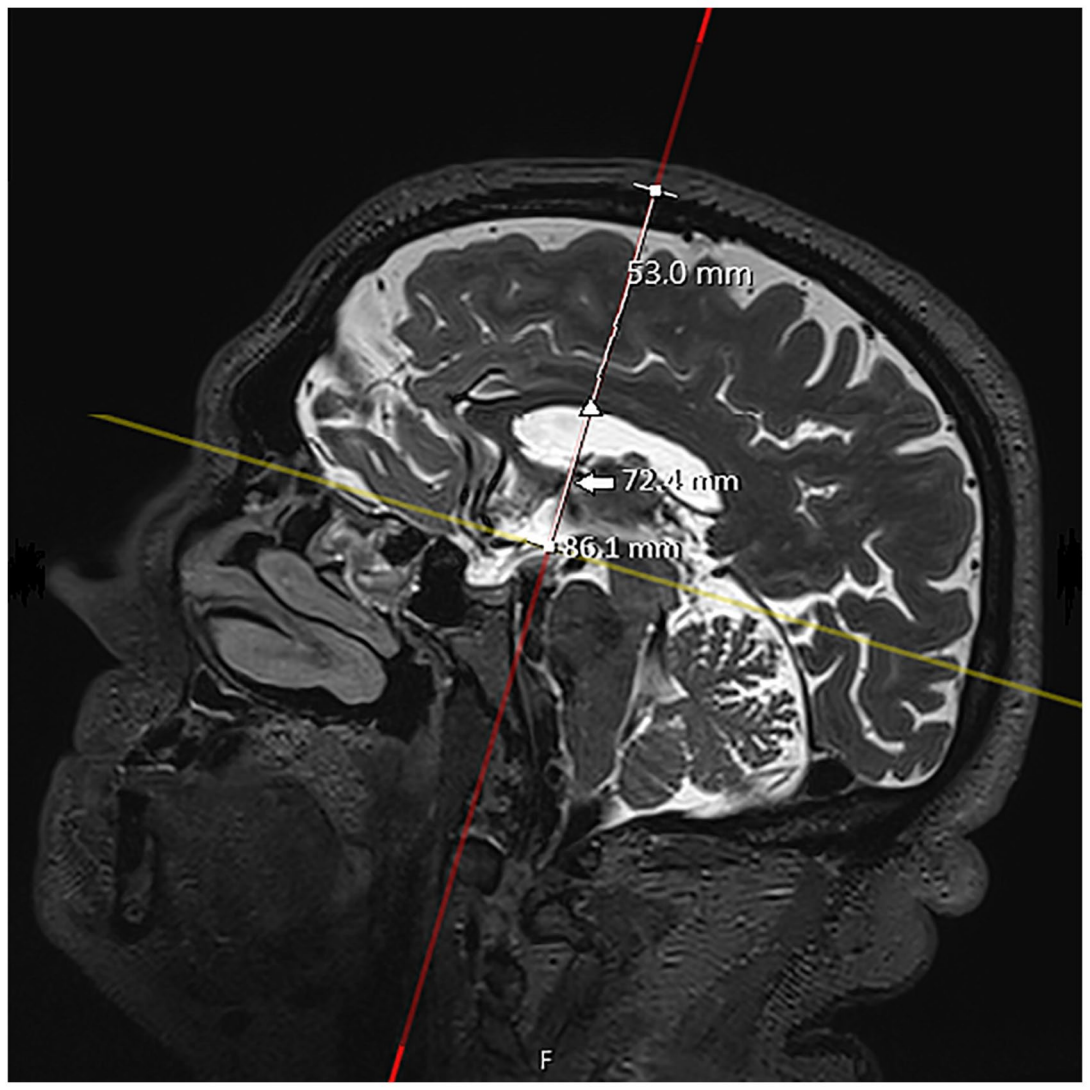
T2 SPACE MRI imaging showing a sagittal view of the brain hemisphere of a non-hydrocephalic patient with Parkinson’s disease. The full white line represents the delineated pathway of the neuroendoscope. The distances between the outer bone lamina (upper square) and the pathway intersections with the tuber cinereum (lower square), interventricular foramen (of Monro) (arrow), and lateral ventricle (arrowhead) were measured. In this patient, the measured depths were 86.1 mm, 72.4 mm, and 53.0 mm, respectively.
